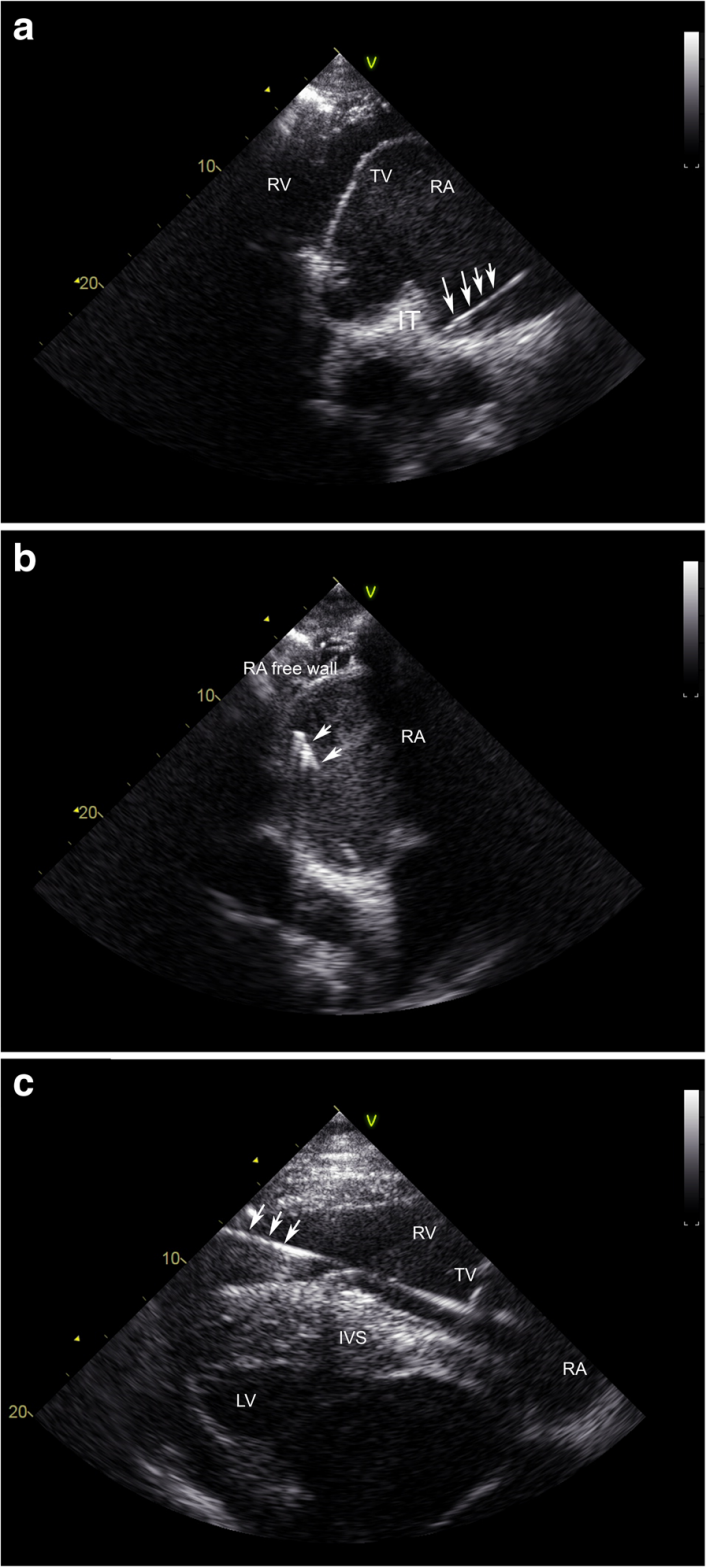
Ultrasound images of the contact MAP electrode (arrow) positioned at the level of the intervenous tubercle (IT) in the right atrium (a), at the level of the right atrial free wall (b) and in the right ventricular apex (c). RA: right atrium, TV: tricuspid valve, RV: right ventricle, LV: left ventricle, IVS: interventricular septum
